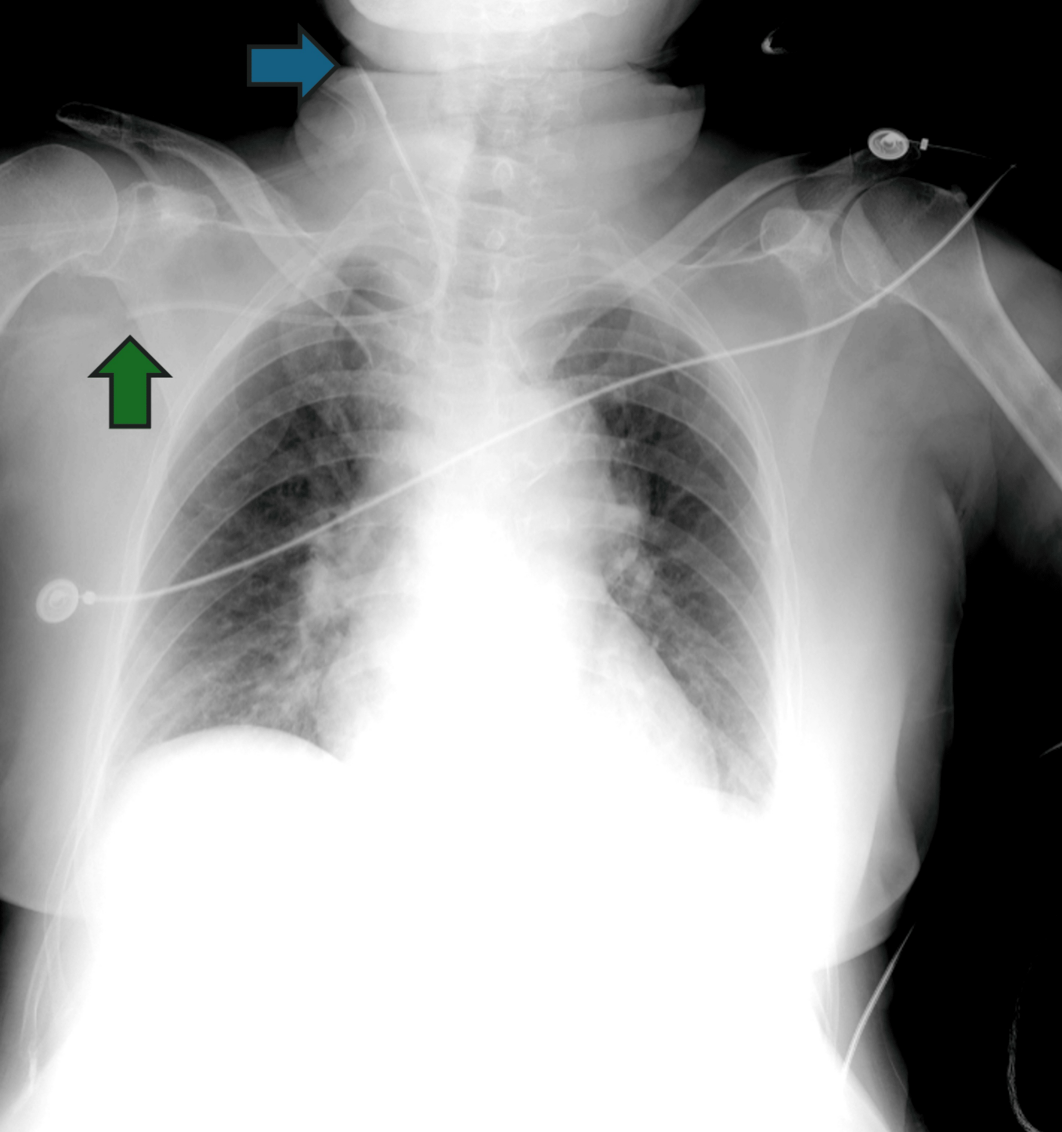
Chest X-ray. The chest X-ray revealed a central venous catheter with the entrance in the right subclavian vein (green arrow) and a misplaced tip (blue arrow).
